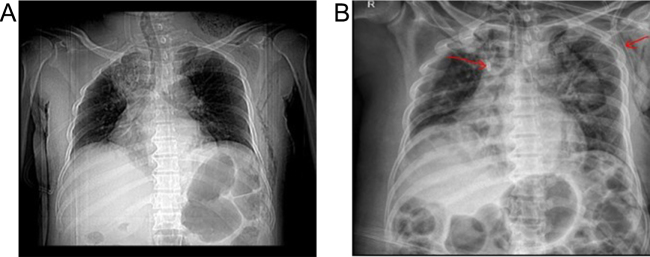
(A) Initial chest X-ray showing a right superior mediastinal opacity. (B) Post-tracheostomy and mediastinal irrigation tube placement showing resolution of right superior mediastinal opacity. Red arrow showing the drainage tube at the superior mediastinum and another in the axillary fold.
